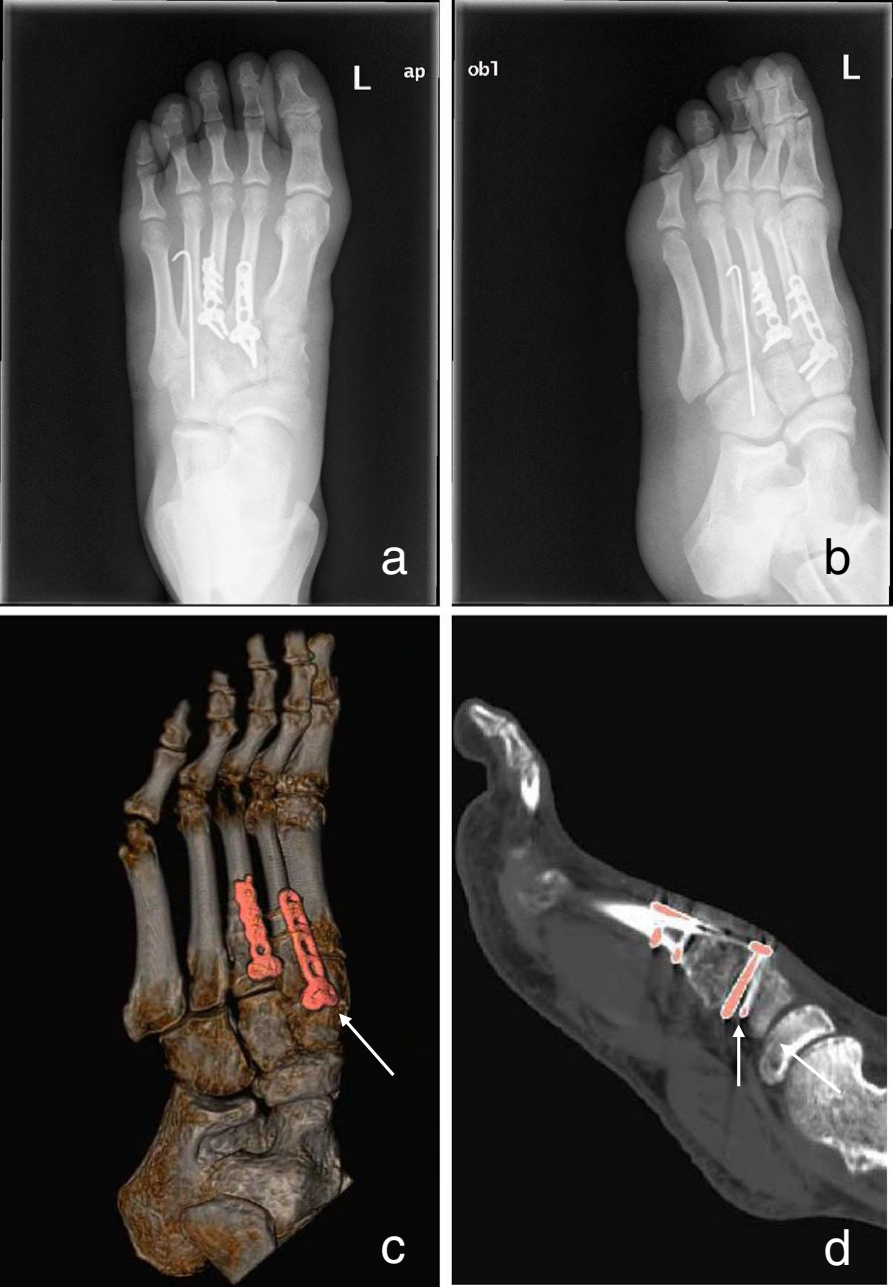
A 36-year-old woman with a Lisfranc injury of Myerson classification B2. In the anteroposterior and oblique plain radiograph (a, b), the internal fixation was placed well. But in the VR mode of CT images, the position of the screws at the second metatarsal base was found like placed into the articular cavity (c), and we checked the image of the articular surface in the MPR mode further and found that one of the screws was place into the articular cavity definitely (d﻿)
